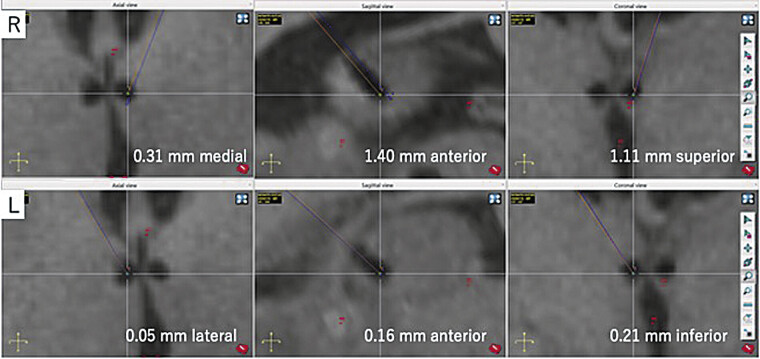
Postoperative electrode location deviations from the preoperative ROSA plan. Postoperative T1-weighted MRI shows electrode tip locations in axial, sagittal, and coronal views. Top row (R): right electrode position with deviations of 0.31 mm medial, 1.40 mm anterior, and 1.11 mm superior to the planned coordinates. Bottom row (L): left electrode position with deviations of 0.05 mm lateral, 0.16 mm anterior, and 0.21 mm inferior to the planned coordinates. The blue line represents the trajectory planned during preoperative ROSA planning. The orange line corresponds to the actual electrode trajectory, reconstructed based on the center of the hypointense region representing the electrode tip.
